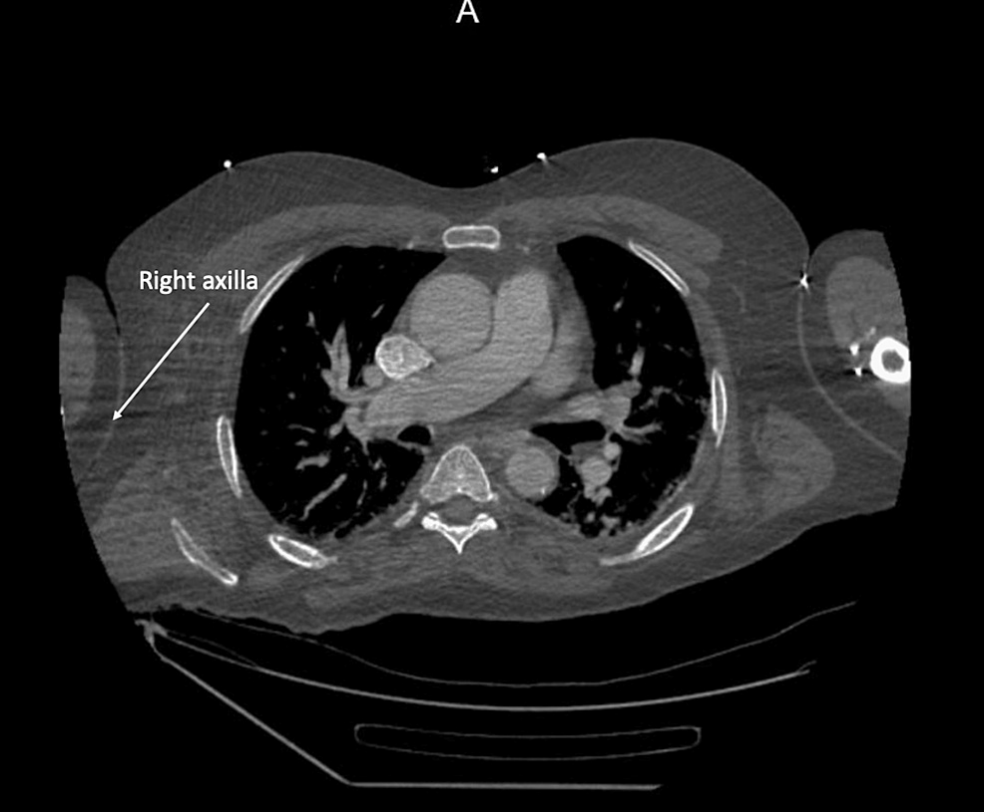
CT scan of the abdomen and pelvis shows nonspecific fat stranding in the right axilla, without evidence of focal fluid collection or subcutaneous gas
